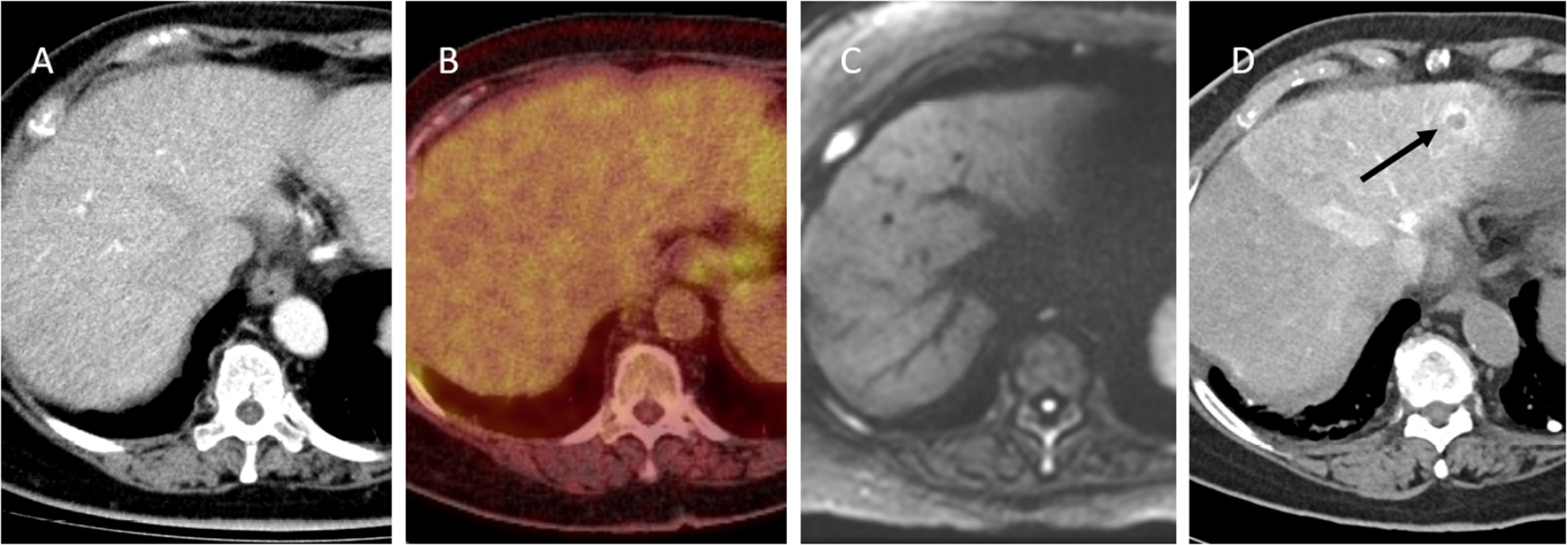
Case with concomitant CRLM: A and B; pre-procedural contrast-enhanced CT and 18F-FDG PET-CT. C: pre-procedural MRI (DWI). D: transcatheter CTHA in arterial phase. An additional ring-enhancing lesion in segment II (arrow) was found intra-procedurally. This lesion was considered highly suspect for CRLM and consequently ablated in the same procedure. This lesion was not detected with the conventional pre-procedural diagnostic modalities
